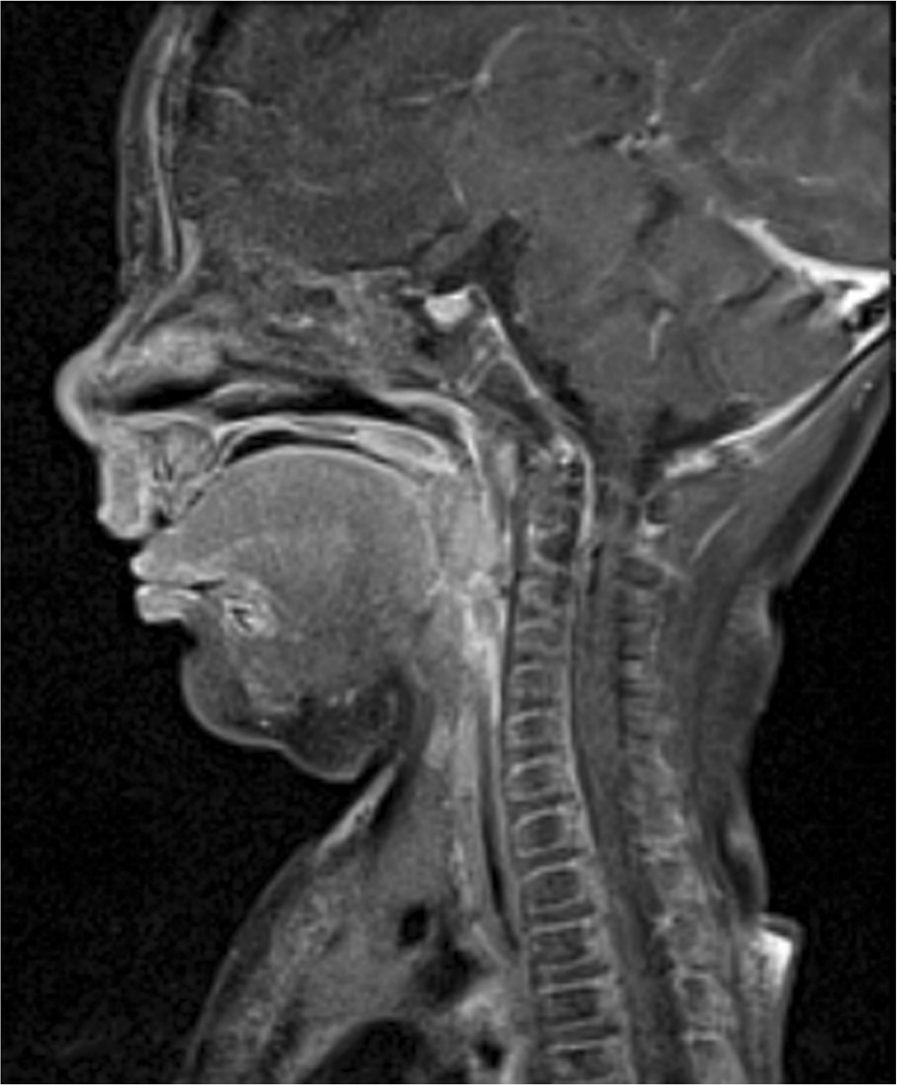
Magnetic resonance imaging of the head and neck region. Sagittal magnetic resonance image (T1-TSE) after contrast showing a pediculated 6.6 x 17.4 x 10 mm muscle-isointense lesion with a moderate uptake of contrast agent at the level of the second and third cervical vertebrae
